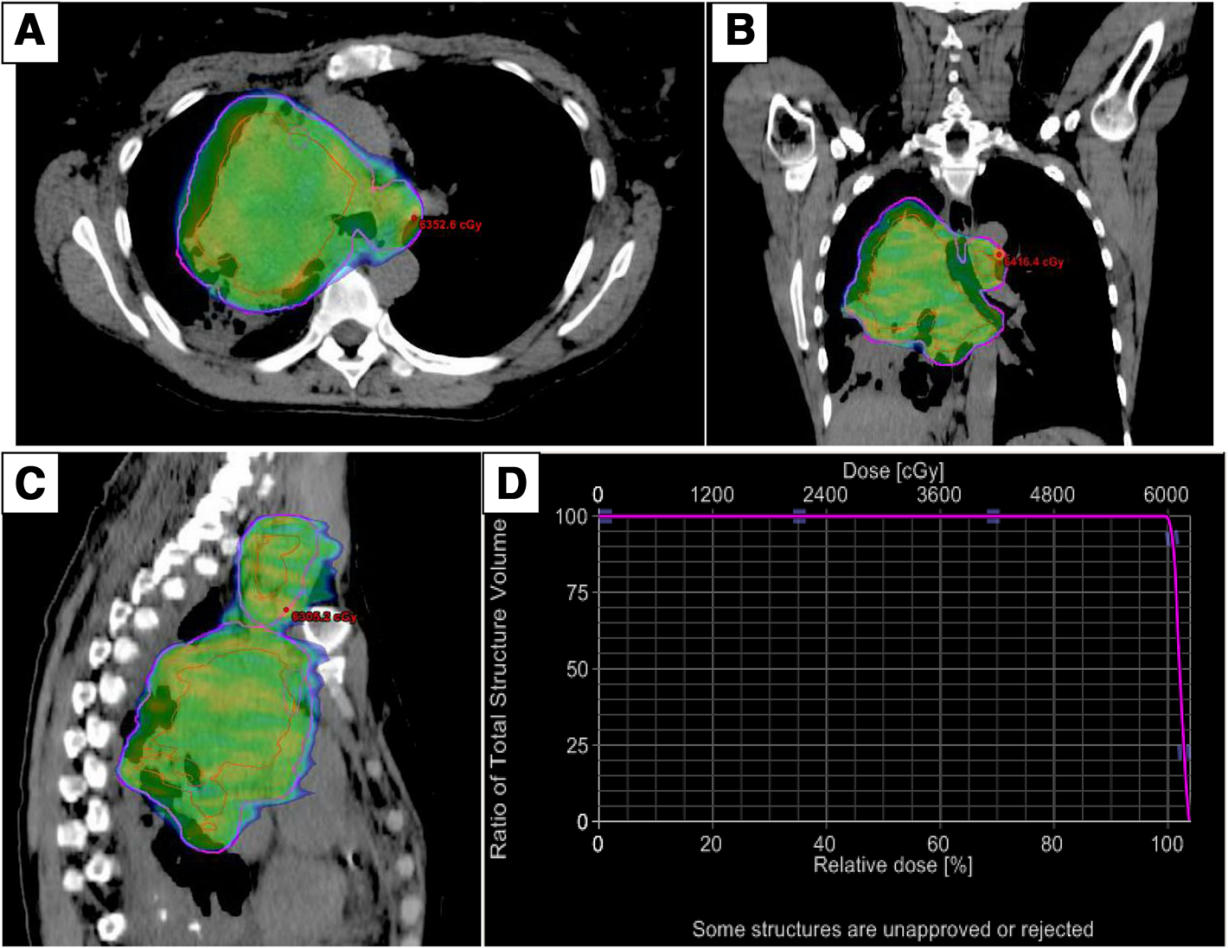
Radiation plan showing 95% isodose coverage (57 Gy). The entire superior vena cava (SVC) is within the planning target volume or high-dose region of radiotherapy. (a) Axial, (b) coronal, and (c) sagittal planning computed tomographic scans. d Dose-volume histogram analysis of SVC (magenta)
